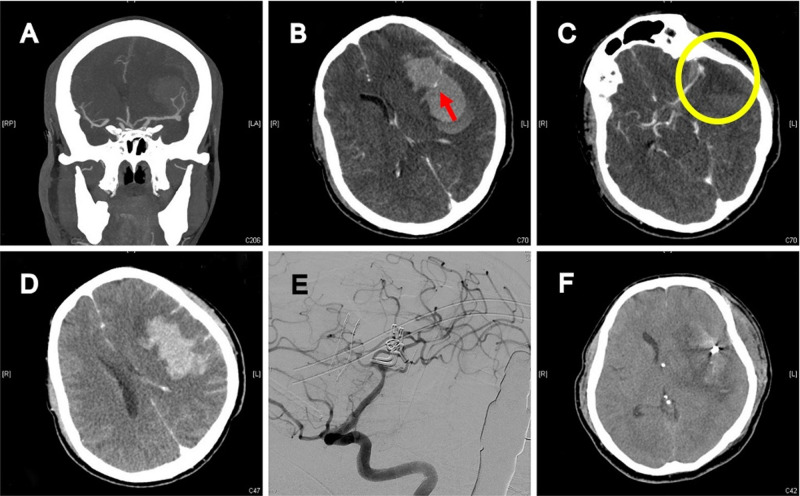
41-year-old female with high-grade aSAH caused by ruptured left MCA aneurysm. (A) Preoperative brain CTA depicted severe aSAH, ICH, and two MCA aneurysms. (B) Intra-hematoma spot sign (red arrow). (C) Perisylvian low density (yellow circle). (D) Distal hematoma. (E) 5 days after craniotomy to remove hematoma and clip two aneurysms; post-operative angiography revealed complete obliteration of aneurysms, well preservation of all MCA branches, and no vasospasm. (F) Delayed progressive mass effect with midline shift.
